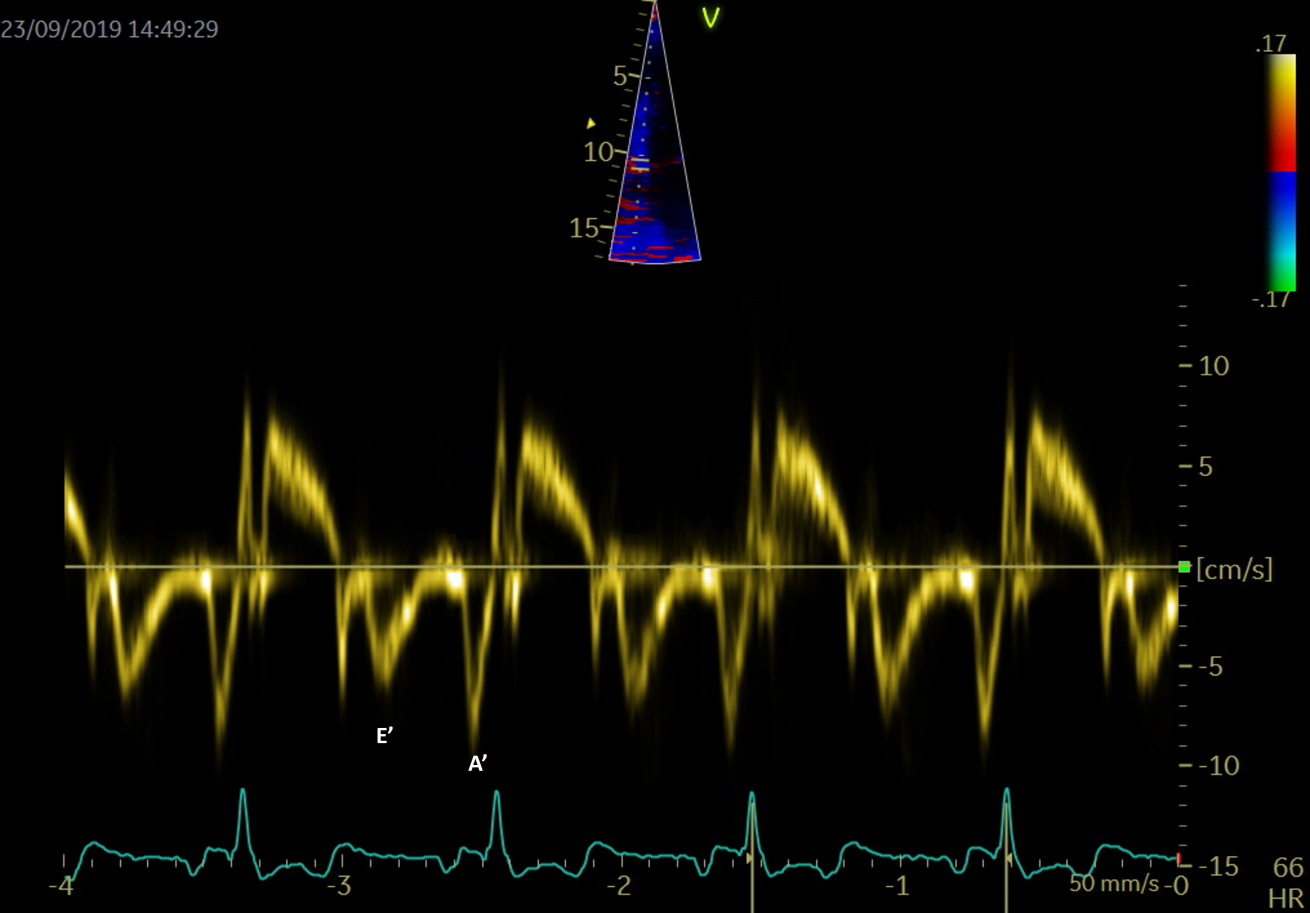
PW Tissue Doppler evaluation at the level of the septal mitral
